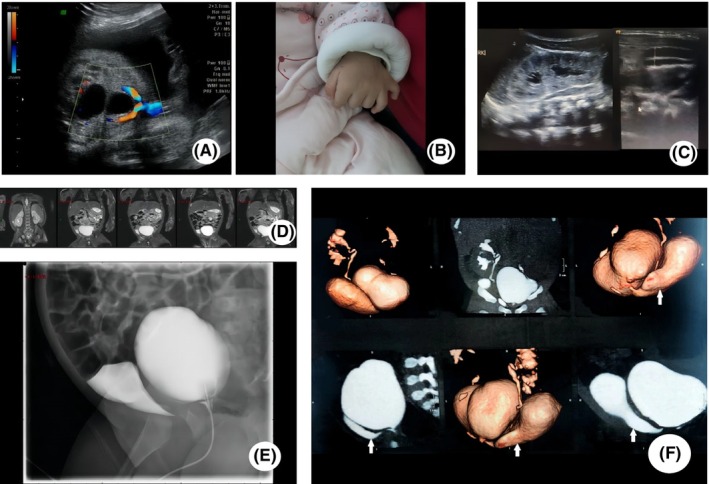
(A) Prenatal ultrasound at 28 weeks gestation showing a cystic structure adjacent to the fetal bladder. (B) Physical examination at birth showing bilateral polydactyly. (C) Repeat prenatal ultrasound showing a right duplex kidney and dilated ureter. (D) MRI at 1 month old showing a cystic mass behind the bladder. (E) Voiding cystourethrography (VCUG) also showing the cystic mass. (F) CT imaging showing the cystic mass and the bladder appeared to unite with a fistulous connection.
